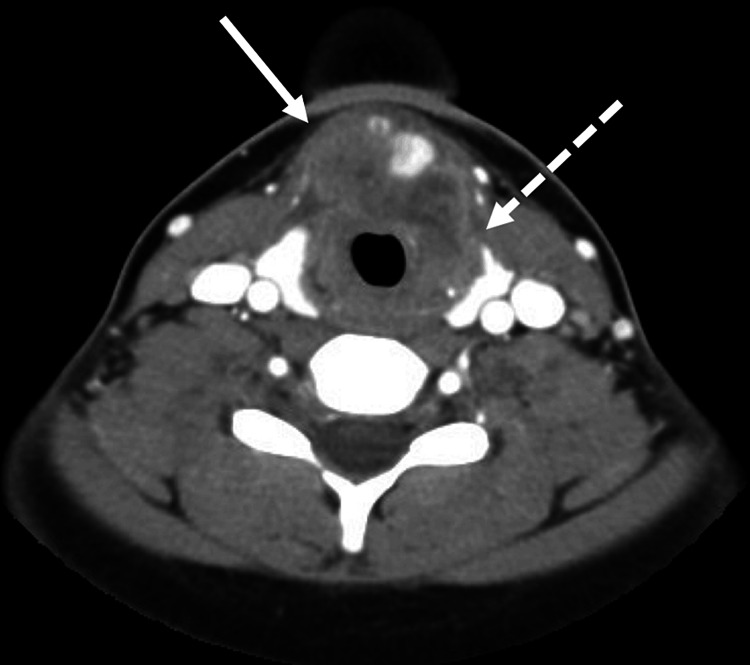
Axial view CT scan of the neck demonstrated the tumor invading the anterior larynx (solid arrow) and thyroid gland (dashed arrow).
